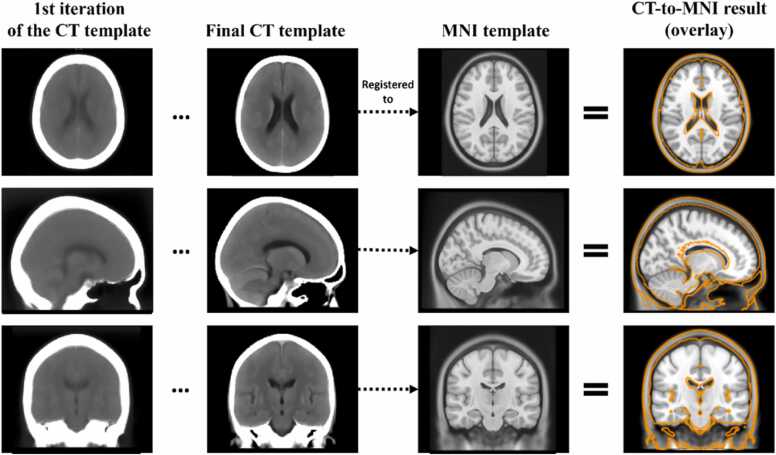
Initial and final CT template, i.e. resulting from the 1st and 7th iteration of the template construction process. The final template is then non-linearly registered to the MNI MRI template. The result of this CT-to-MNI registration is shown as the orange contour, overlaid on the MNI template image for verification of anatomical correspondence. This transformation was then used to map the brain regions, originally parcellated in MNI space, to the CT template space.
